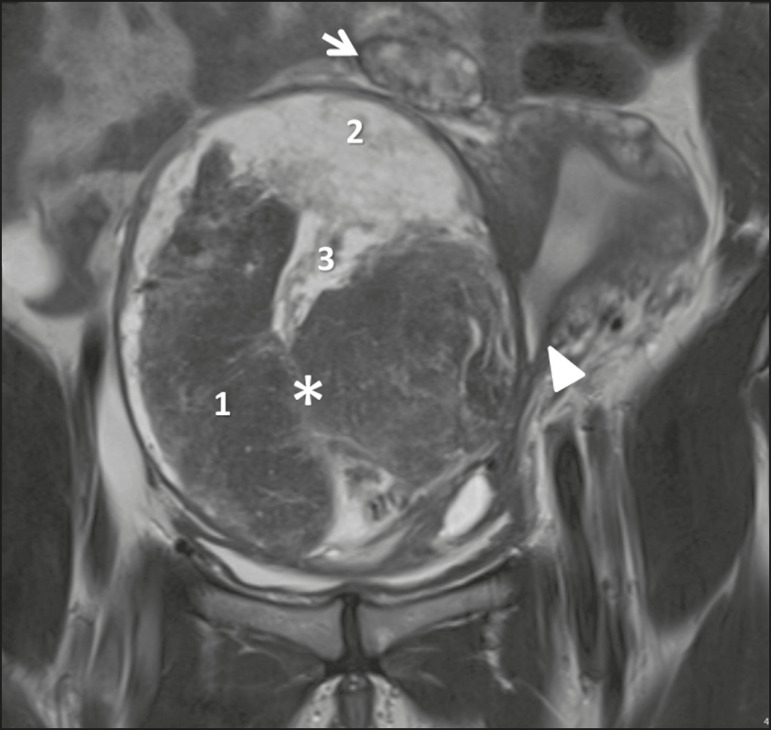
Leiomyoma of the broad ligament with hydropic degeneration in a 43-year-old woman. Coronal T2-weighted imaging showing a markedly heterogeneous tumor (asterisk) with an inferior solid component, displaying isosignal to the muscle in T2 (1) and a superior part comprising multiple large cystic areas showing high T2 signal (2), with cord-like solid components (3), which is clearly demarked from the right ovary (arrow) and the uterus (triangle).
